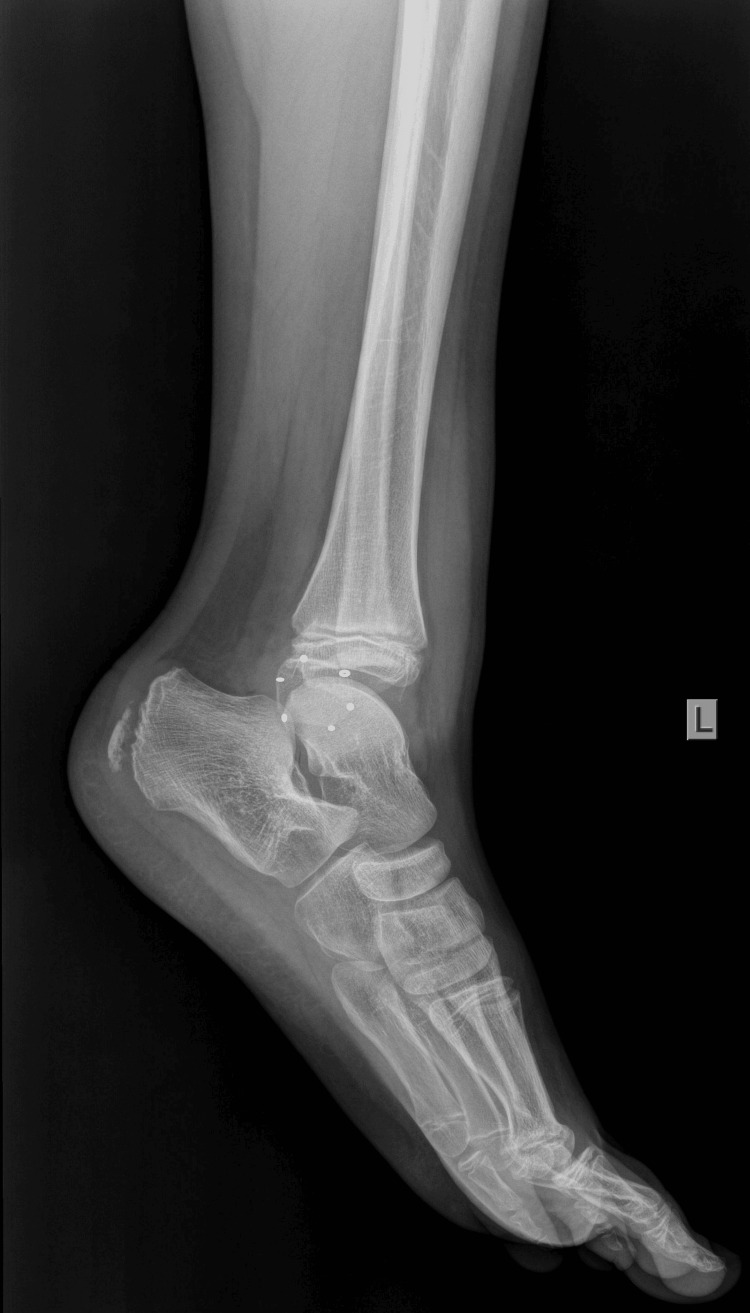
Reviewing the lateral X-ray with the dotted line outlining the distal fibular epiphysis that is completely displaced in relation to the fibular metaphysis.
